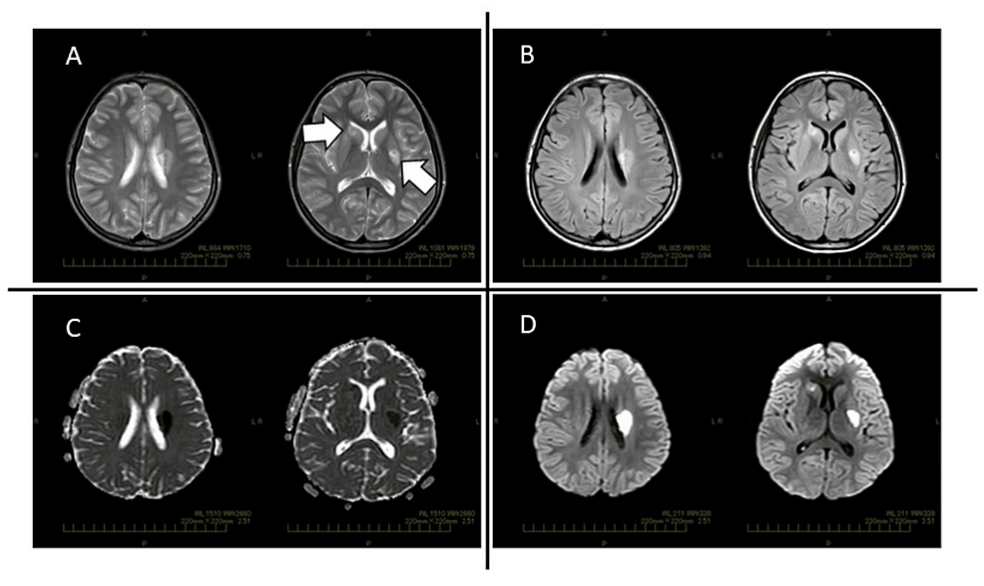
Plain MRI of the patient's brain in our hospital Plain MRI of the brain indicated high-signal areas in the right caudate nucleus, internal capsule and left basal ganglia when applying T2-weighted, fluid-attenuated inversion recovery (FLAIR), and diffusion-weighted imaging (DWI); and low signals in the same regions in an apparent diffusion coefficient (ADC) map. (A) T2-weighted images, (B) FLAIR, (C) DWI, (D) ADC map. White arrows point to the lesions.
